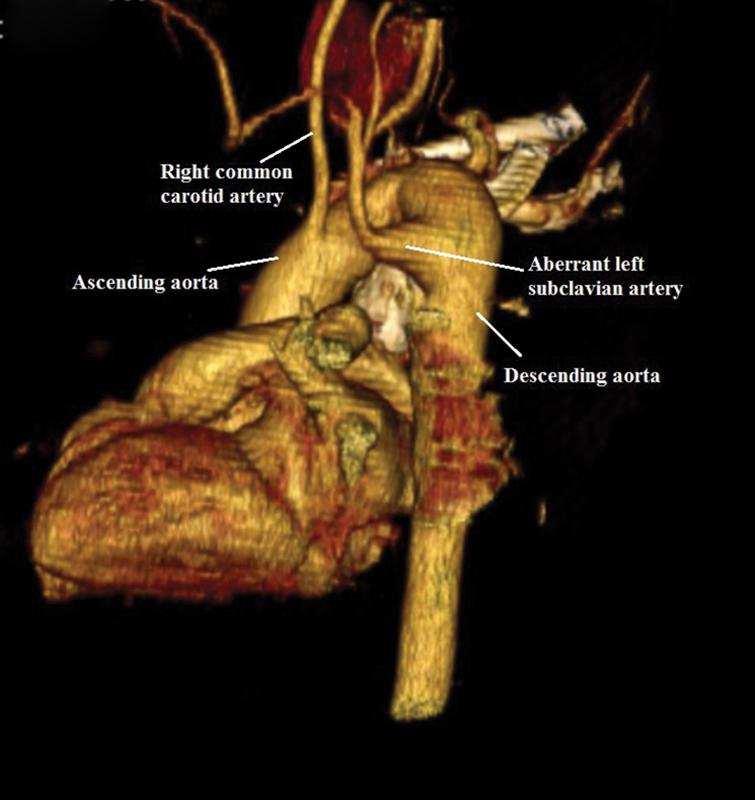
3D computed tomography angiography image. Right aortic arch with aberrant left subclavian artery. 3D, three-dimensional.
